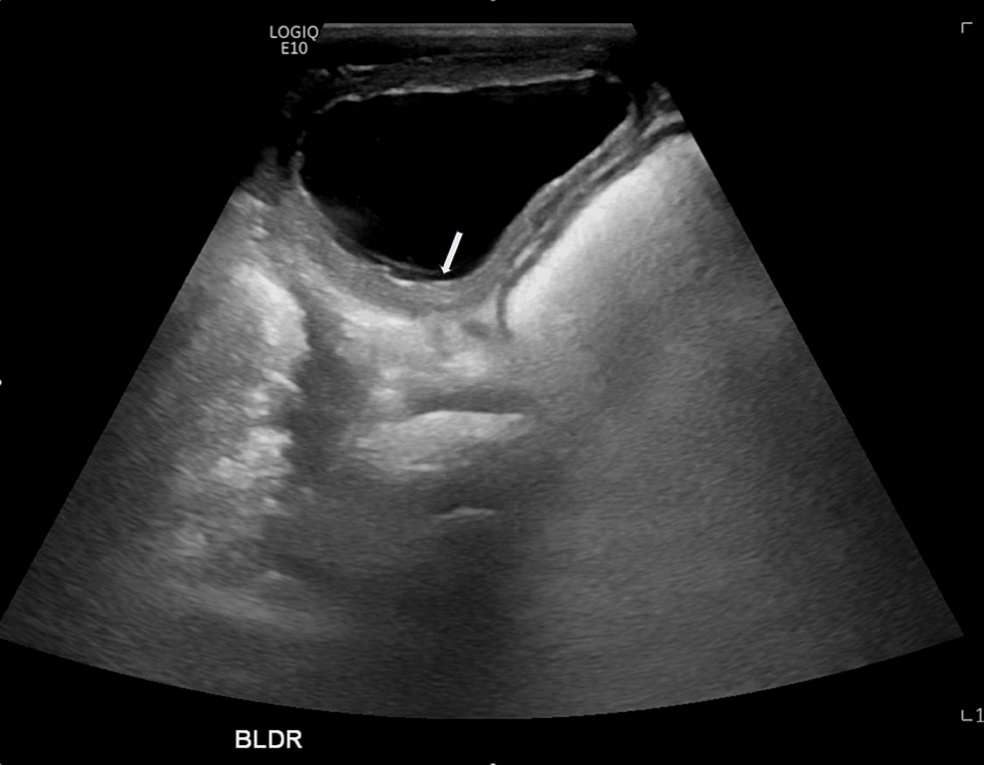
Ultrasound showing thickened bladder wall.
